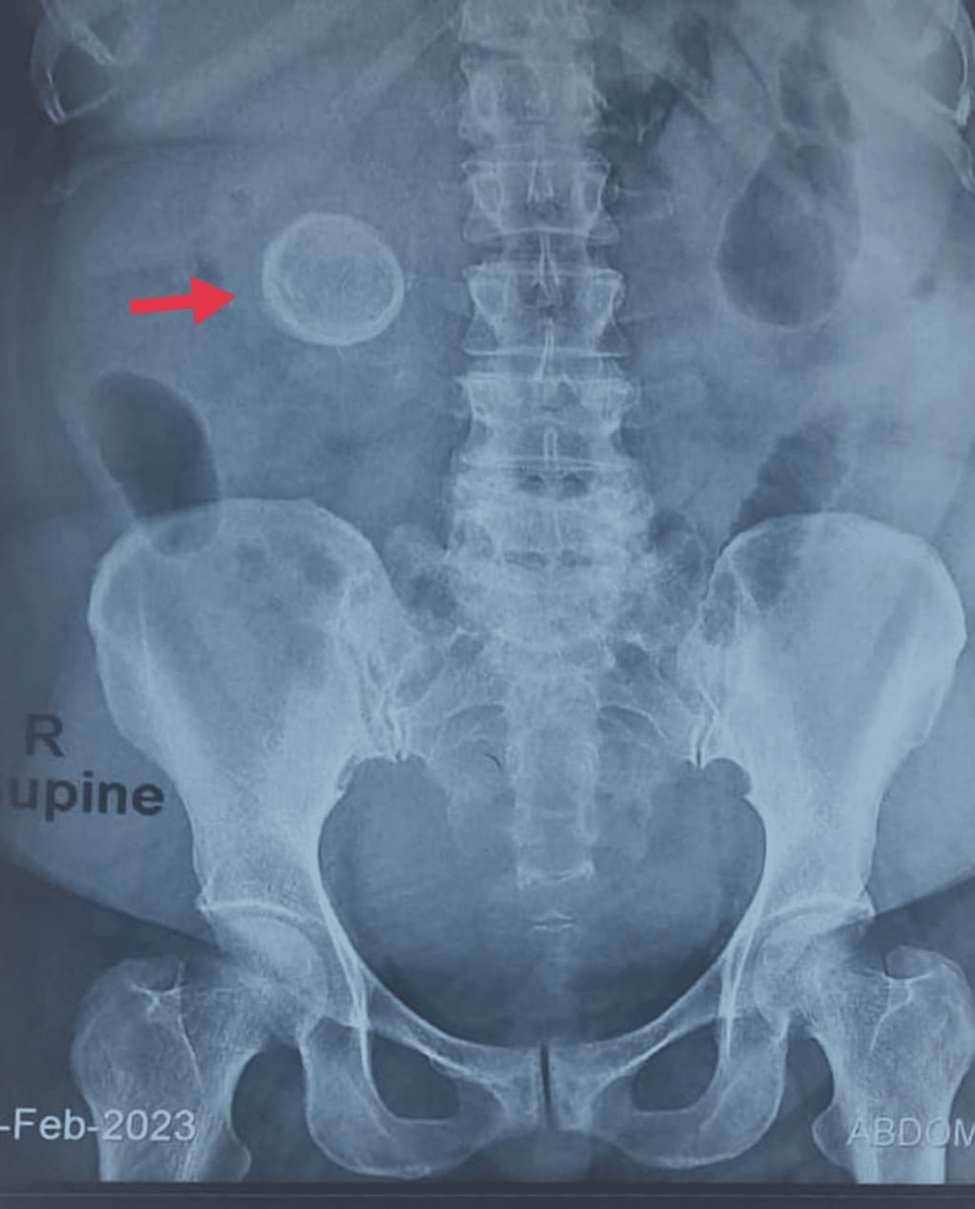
Plain x-ray of the abdomen depicting an oval radio-opaque shadow in the subhepatic region.
